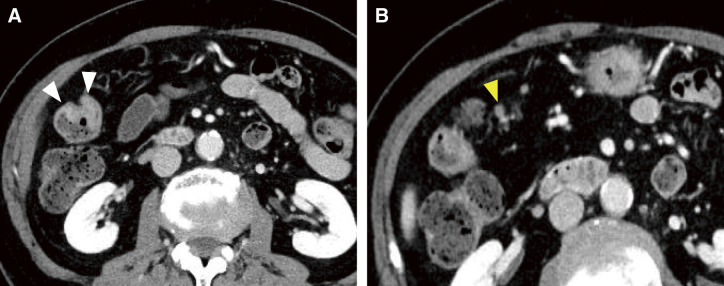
Contrast-enhanced computed tomography images. Images show a tumor located near the hepatic flexure (white arrowheads) (A) and an enlarged lymph node near the tumor (yellow arrowhead) (B).
